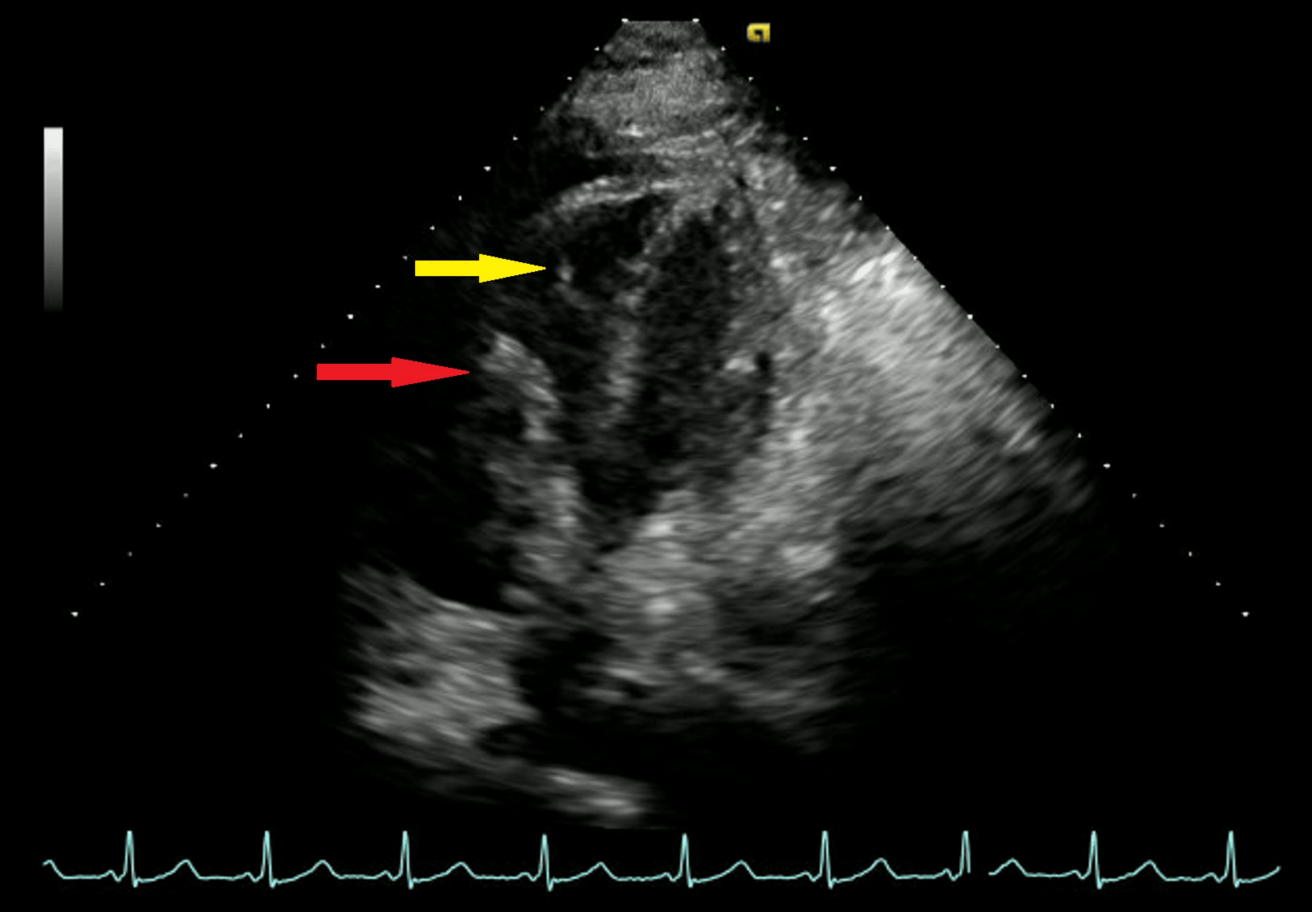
Apical view of transthoracic echocardiogram showing a right atrial thrombus-in-transit (red arrow) extending into the right ventricle (yellow arrow).
